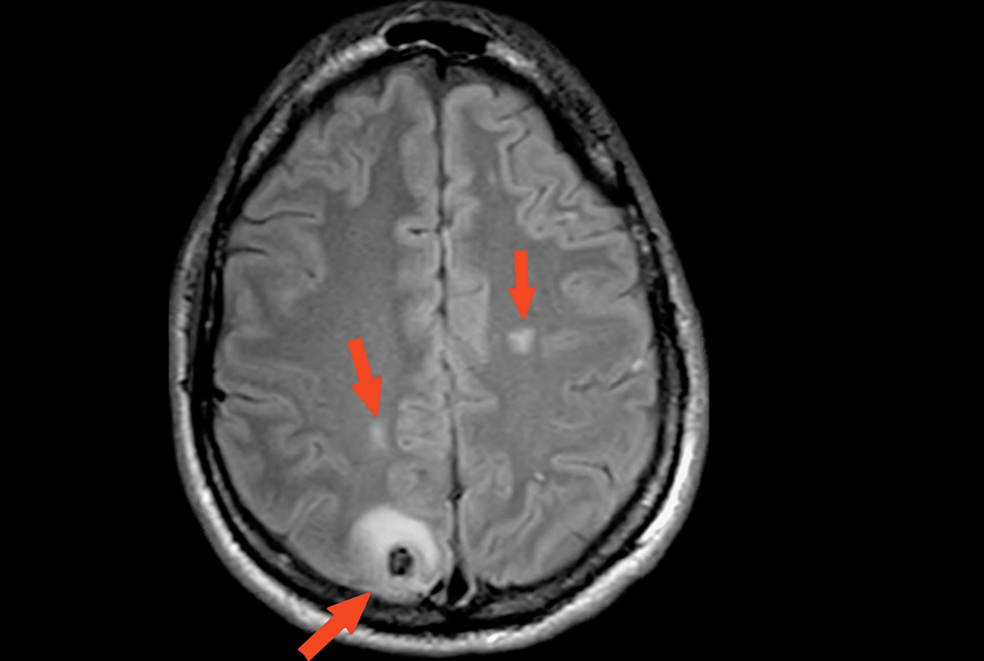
MRI Brain T2 FLAIR, hyperintensive lesions, largest located right posterior parietal lobe with perifocal edema consistent with blood product. MRI, Magnetic resonance imaging
